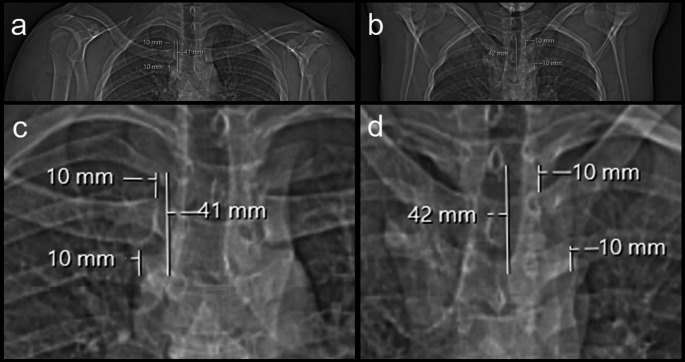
Scan length planning on scout views. Examples with arms-down (a) and with arms-up (b) as well as magnified scout views with arms-down (c) and arms-up (d) The CT scan length is planned on the magnified scout views as they allow for better delineation of cortical contour of the clavicle. The starting point of the CT scan is 10 mm above the superior contours of the medial clavicle, the endpoint is 10 mm below the inferior contour of the MCE. In these two cases, the planned scan lengths were 41 mm and 42 mm, respectively
